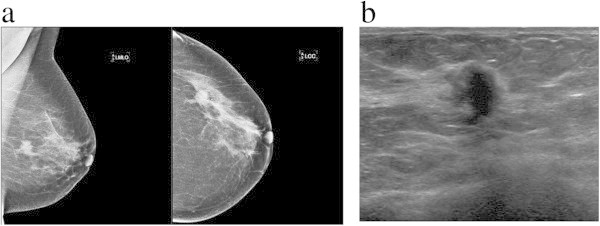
48 year old woman, with positive family history for breast cancer, presented with a palpable lump on the left breast, finally diagnosed as ductolobular carcinoma with a ≤ 20% proportion of the lobular component. a) Mediolateral oblique and craniocaudal mammograms show an irregular and spiculated mass. A comet-tail like projection arises from the anterior and posterior margins and a discrete retraction around the lesion can be seen. b) Ultrasound of the left breast demonstrates an irregular, hypoechoic and vertically oriented mass, with echogenic halo at 3 o’clock position.
